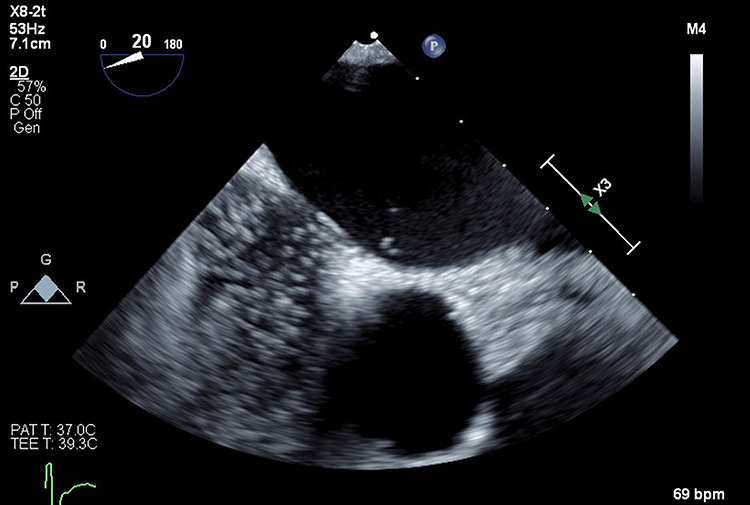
Transesophageal echocardiography. Agitated saline study with bubbles crossing from right atrium to left atrium through PFO.
